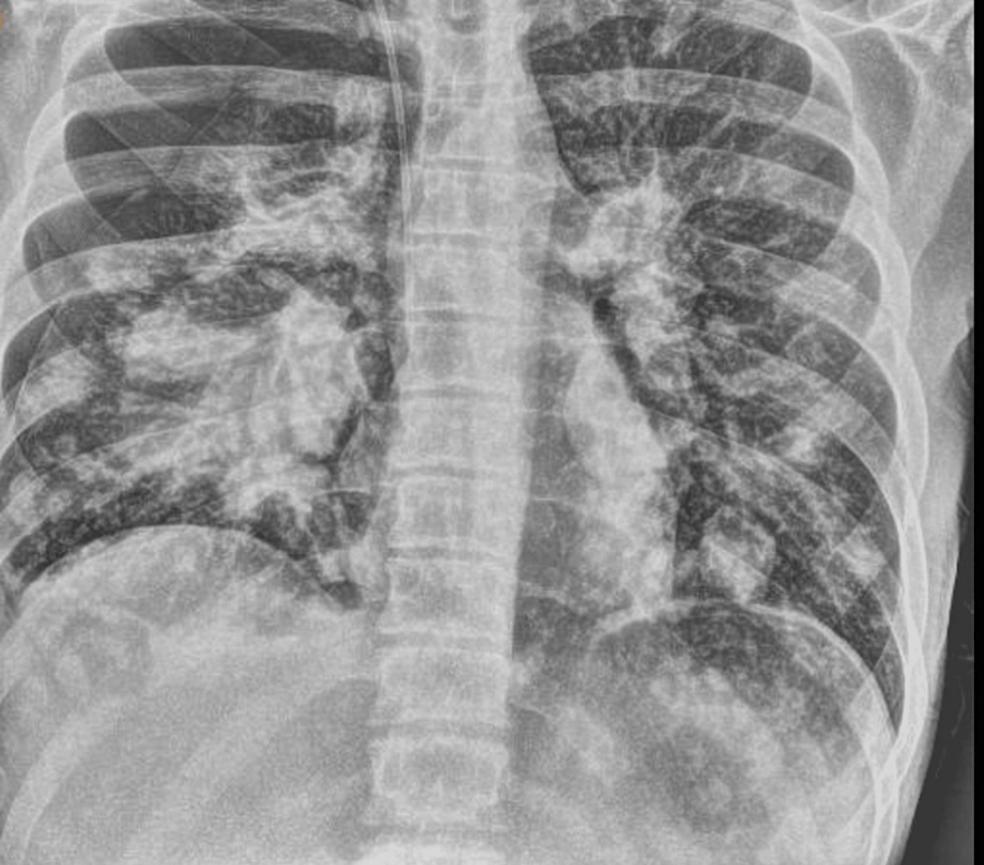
Chest X-ray showed pneumonitis most likely due to radiation.
